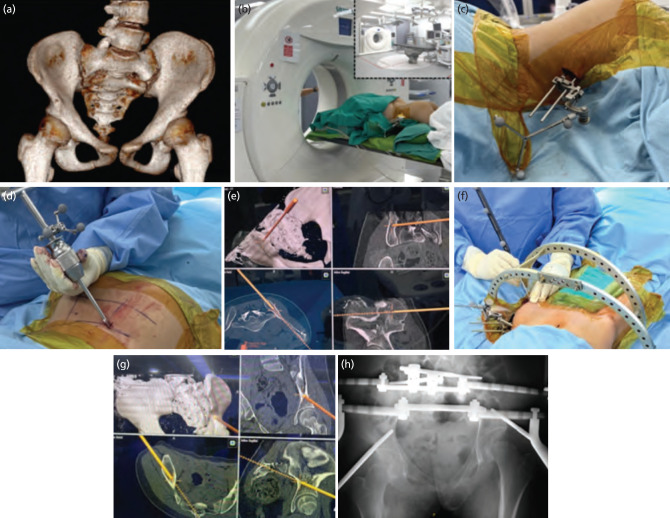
(a) 3D reconstruction CT image of pelvis show pelvic rotation. (b) CT scans were performed in the operating theatre to calibrate the navigation system. (c) Two 3.5mm Schanz pins were inserted into the left iliac crest to secure the navigation tracker. (d) The navigation tracker was attached to the T-handle to guide the insertion of the HA pin. (e) Images showed insertion of the HA pin into the posterior iliac wing under navigation guidance, with a user interface displaying pelvis in 3D, coronal, axial and sagittal views. (f) The navigation probe was used to determine the trajectory and optimal position of the supra-acetabular pin. (g) Images on the user interface show the position of inserted supraacetabular HA-pin. (h) Pelvic radiograph showed final pelvic pin placement in HPT.
